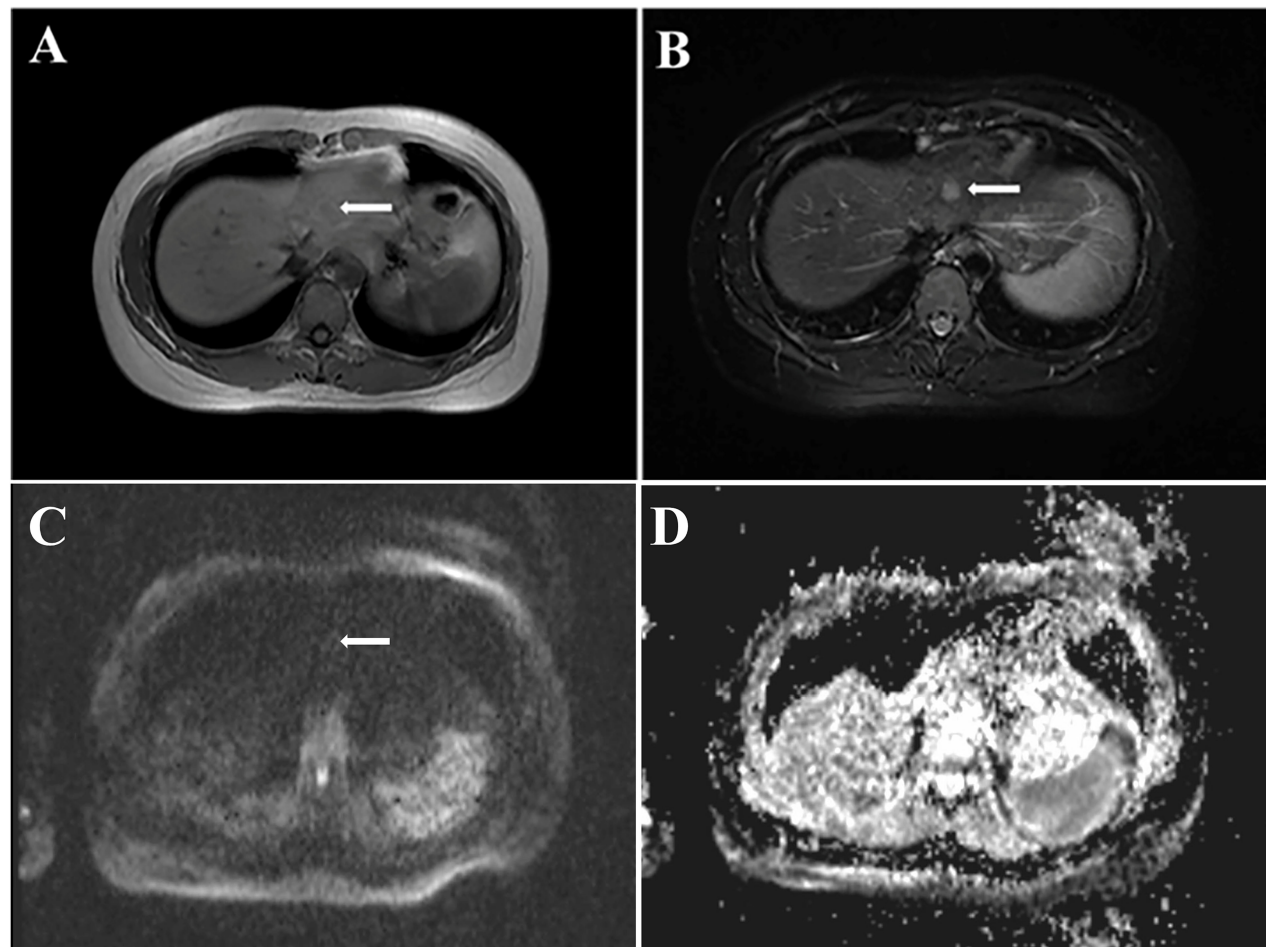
Magnetic Resonance Imaging showed a 1.1×1.3 cm lesion in the left lobe of liver. (A) low signal intensity on T1-weighted images; (B) high signal intensity on T2-weighted images; (C) ring enhancing on DWI image; (D) ADC image of the lesion (ADC value: 800).
